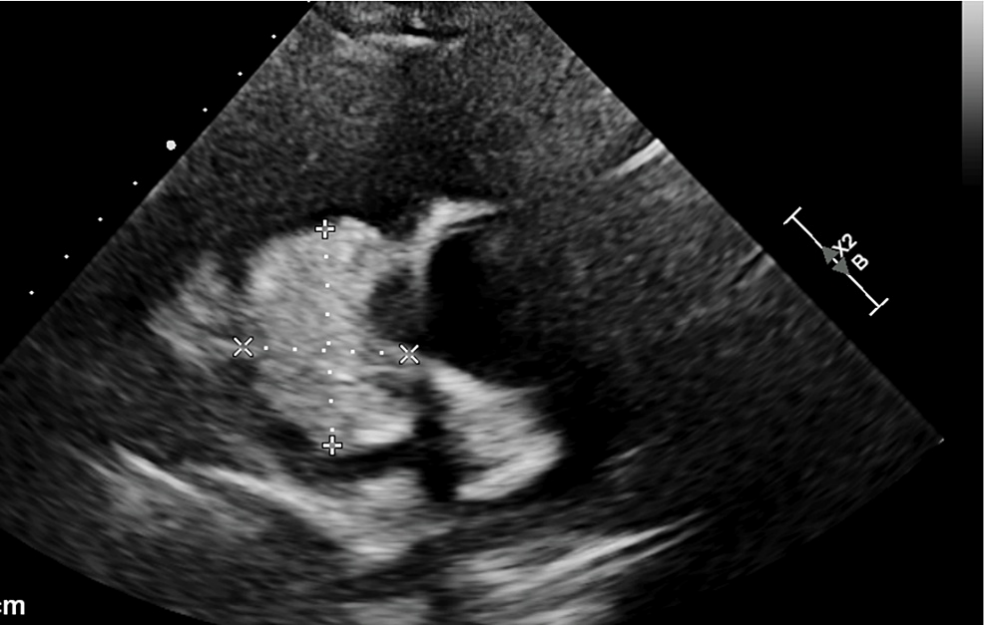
TTE parasternal short axis view at the level of the aortic valve depicting a large 4.3 x 4.0 cm spherical mass arising in the right atrium TTE: transthoracic echocardiogram
